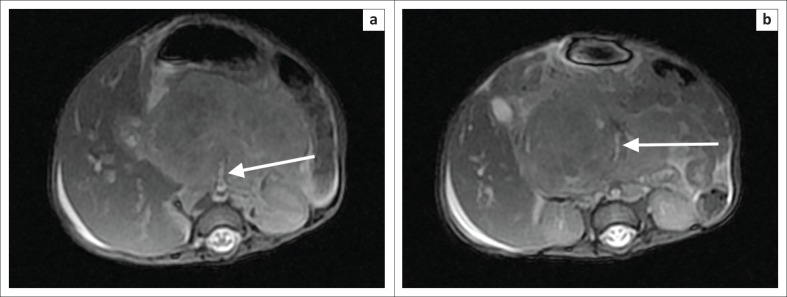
Axial T2-weighted (T2W) magnetic resonance images showing the pancreatic head mass with intermediate signal intensity and encasement of the superior mesenteric artery (white arrow) in (a) and (b) – sandwich sign.
